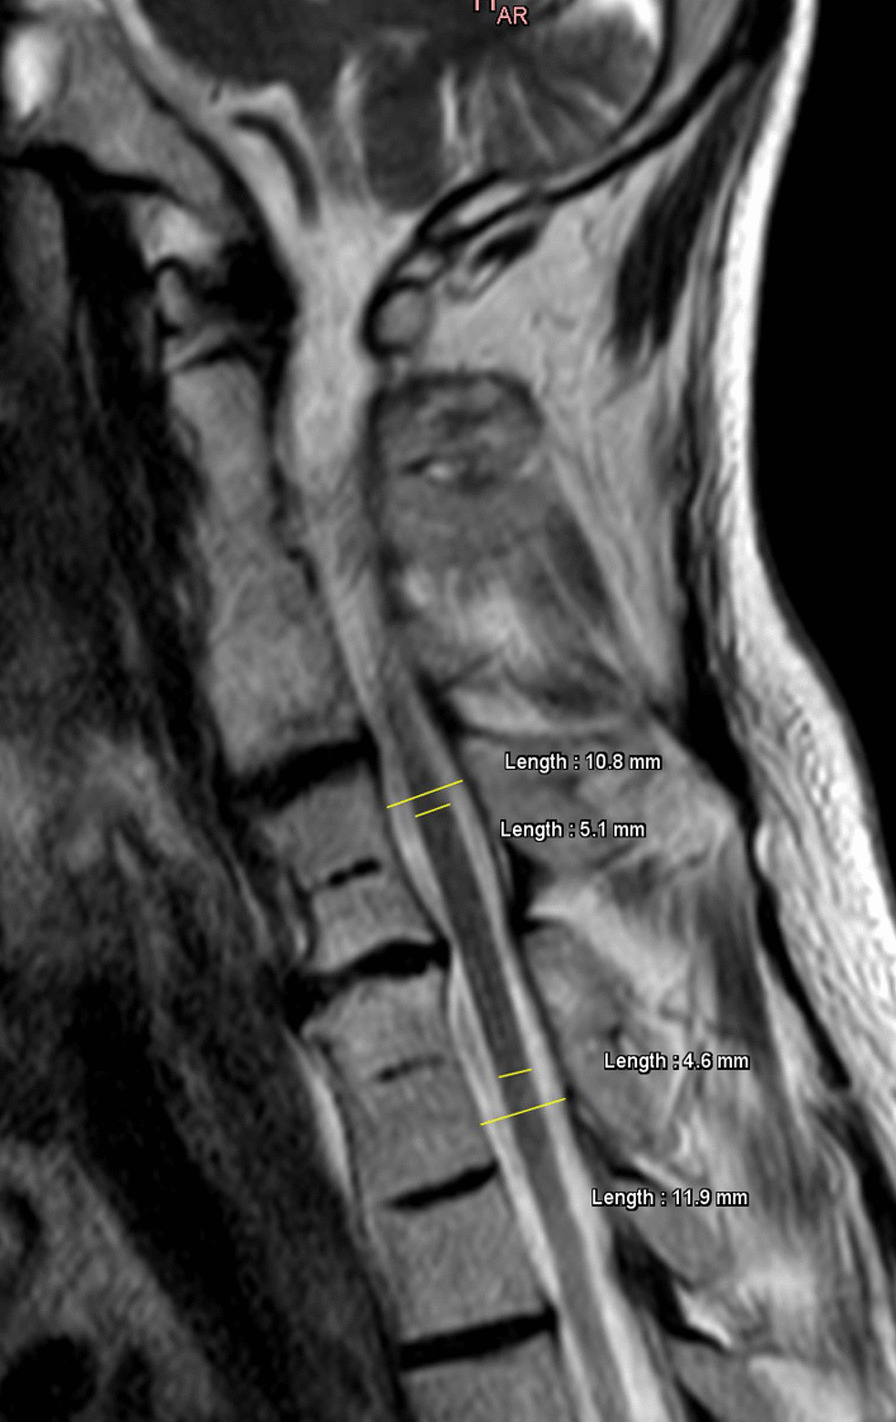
Cervical spine T2-sequence magnetic resonance imaging showing the calculation of spinal cord occupation ratio index. The spinal cord and canal diameters are measured immediately above and below the area of degenerative pathology
